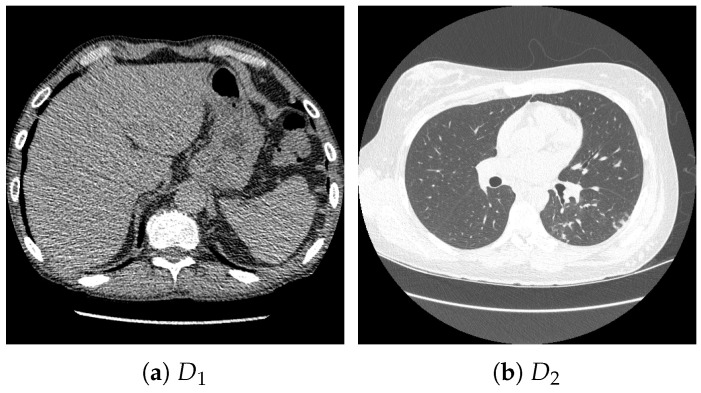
Examples of chest CT images on the subset from the RICORD dataset: (a) $D_{1}$ and (b) $D_{2}$.
